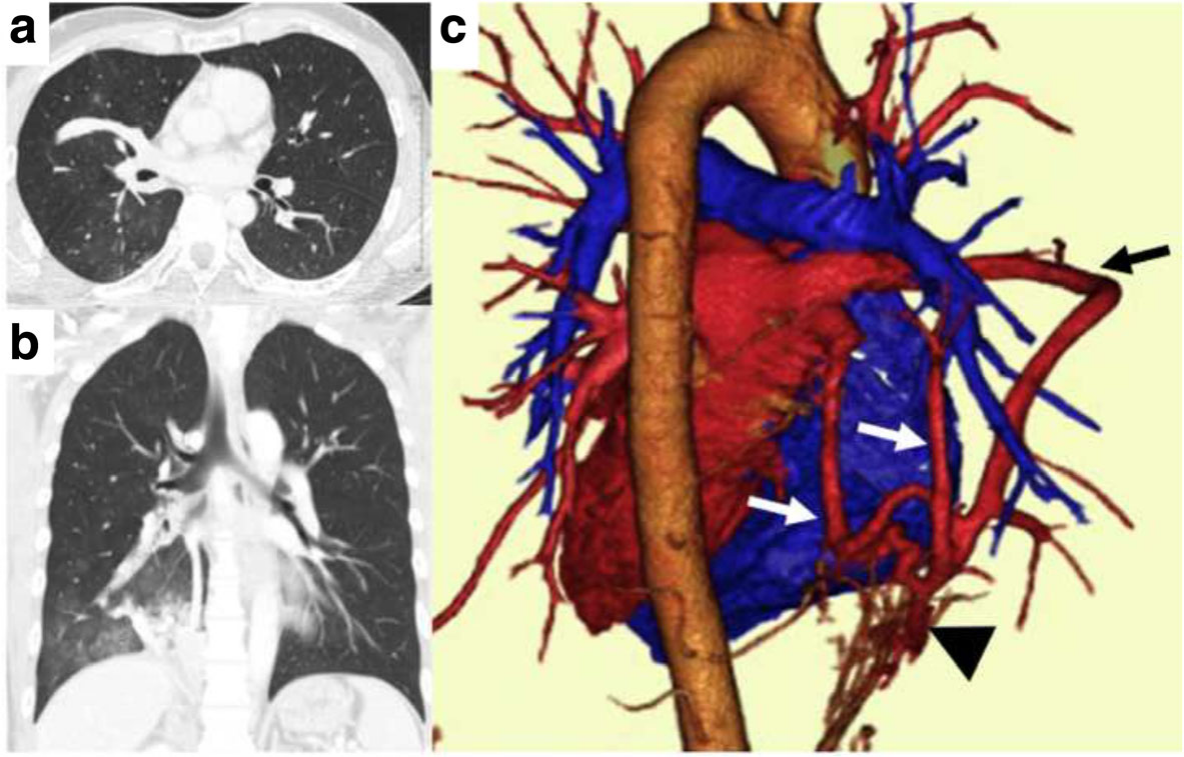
Multiple anatomical abnormalities demonstrated by CT images and their 3D reconstruction. a Axial-plane CT reveals the presence of an arched vein contiguous with the superior and inferior pulmonary veins. b Coronal-plane CT shows a tracheal bronchus. c Three-dimensional reconstructed image demonstrates anomalous venous drainage (*arrows*) and a systemic arterial supply from the abdominal aorta through the diaphragm (*arrowhead*). Note that there are three main anomalous venous arches penetrating the right lung almost regardless of the lobes and connecting to one another at the bottom of the lung. Venous drainage from the upper lobe is preserved and relatively normal. Numerous abnormal arteries are coursing from the systemic circulation at the diaphragm; there might be a direct connection between these arteries and the anomalous venous system
